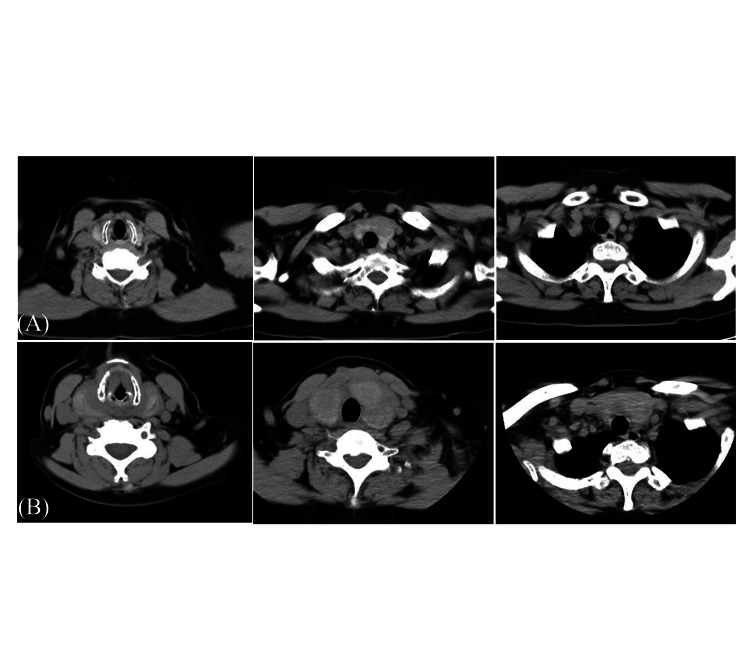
CT images obtained before (A) and after (B) FNAC (A) Pre-FNAC CT shows diffuse thyroid enlargement with an LDA within the gland. (B) Post-FNAC CT demonstrates marked enlargement of both thyroid lobes. The internal attenuation values exhibit heterogeneous reduction, and a slightly hyperattenuating soft tissue area, approximately 50 HU, is observed surrounding the thyroid. FNAC: Fine-needle aspiration cytology; LDA: Low-density area
